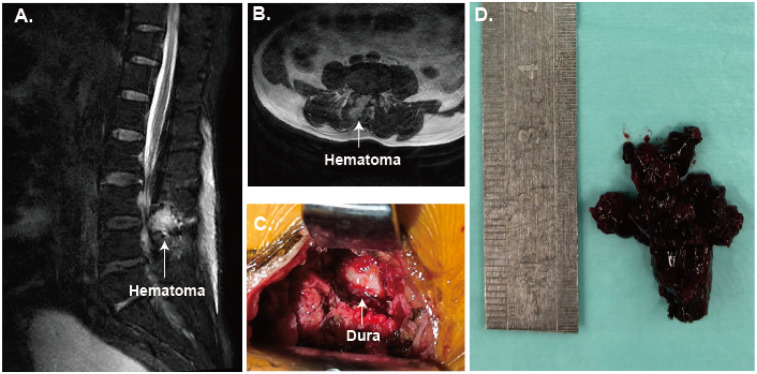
Postoperative hematoma imaging following UBE procedure. Postoperative MRI revealed epidural hematoma causing compression of the thecal sac (A,B). Upon surgical re-exploration, an epidural hematoma compressing the dural surface was identified (C) Photograph of the evacuated hematoma (D).
